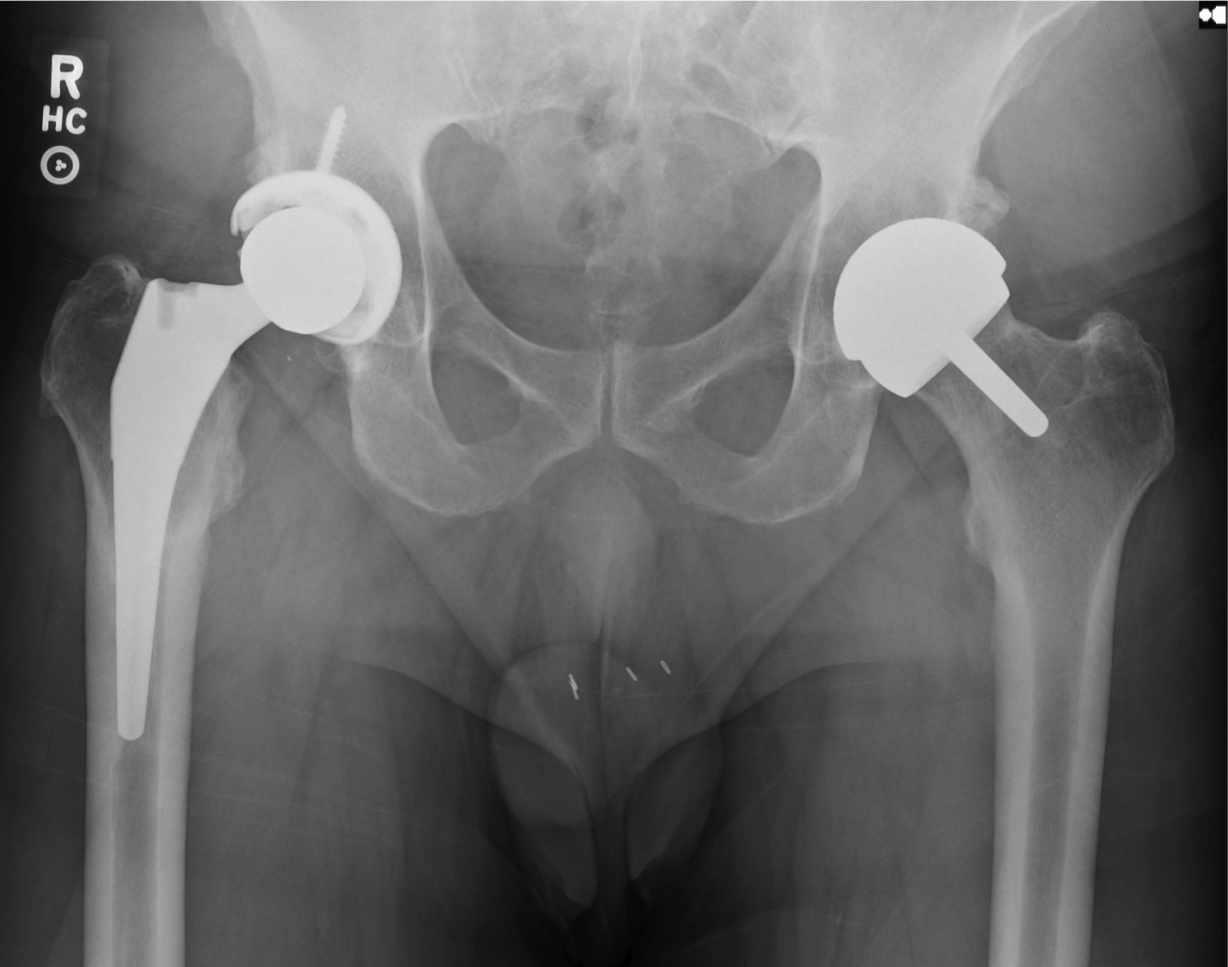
Anterior–posterior radiograph of bilateral total hip arthroplasty.
